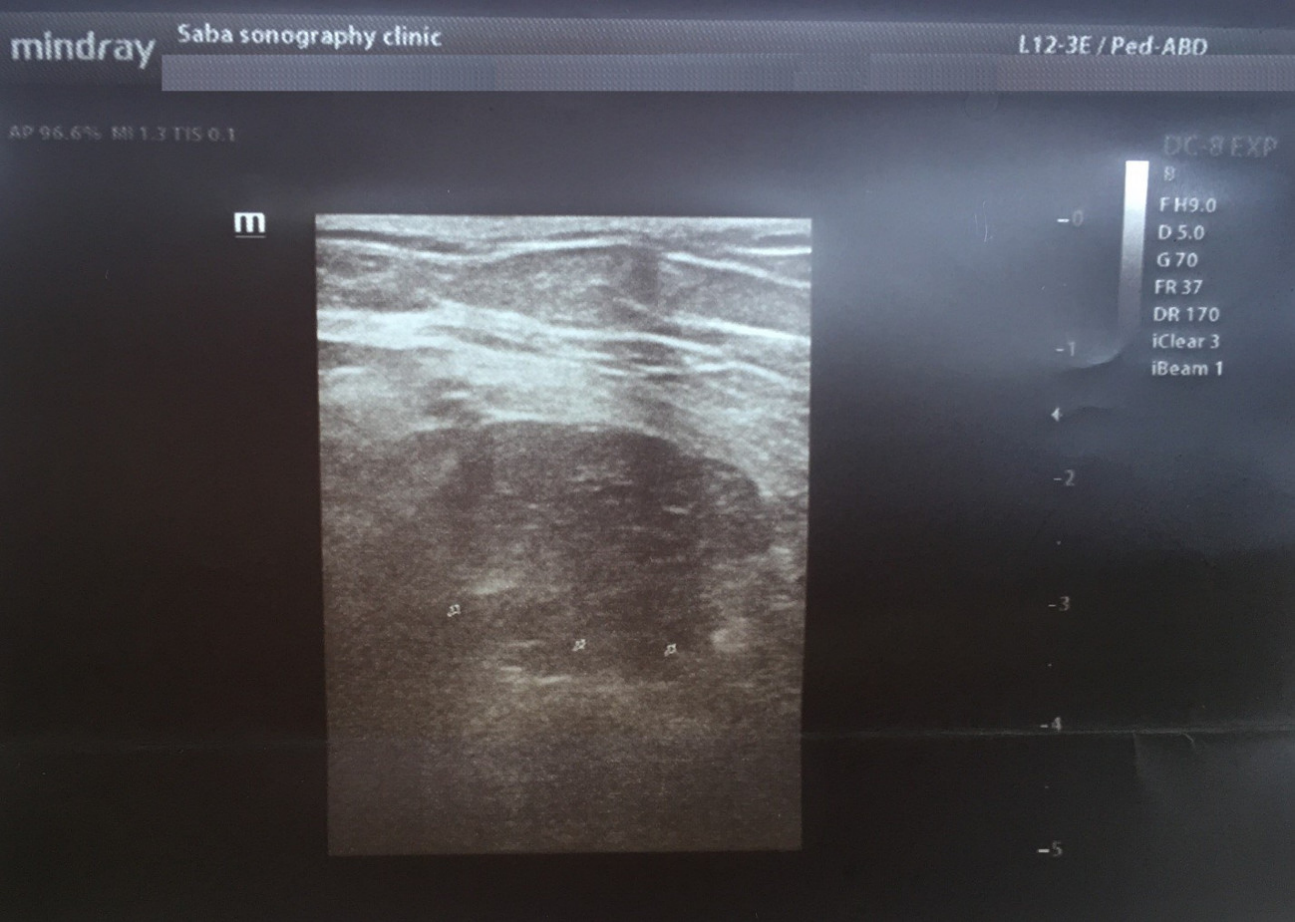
Ultrasound of the thigh revealed an encapsulated multi-cystic lesion with an indistinct margin (arrowheads) or a well-defined hyperechoic wall (arrow) corresponding to a calcified, non-vital hydatid cyst
